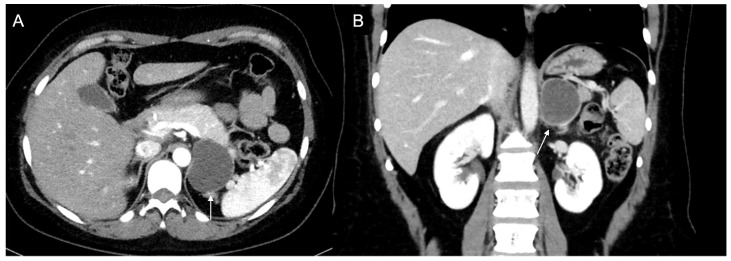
Man 60 years old with left adrenal incidentaloma. Hypodense mass (arrow) without contrast enhancement on CT (A,B), typically benign, stable in follow-up.
